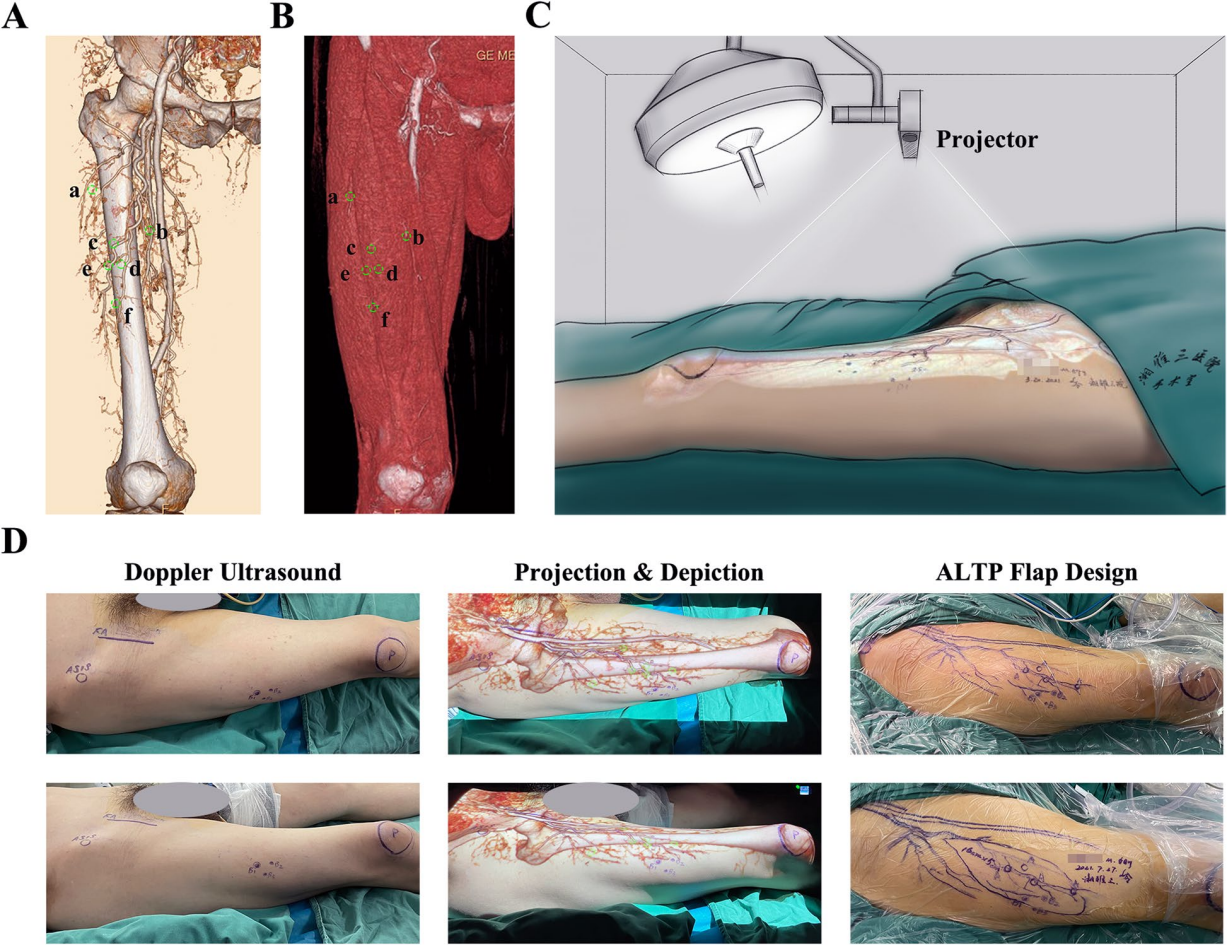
Augmented reality (AR) for ALTP flap design. A The points a-f were marked on the 3D CTA images. B The points a-f were marked on the muscle surface. C The marked images were imported in the portable projector, and then mapped onto the thigh surface before operation. D Doppler ultrasound and AR were used to depict the perforators and emerging points, to facilitate the ALTP flap harvest
